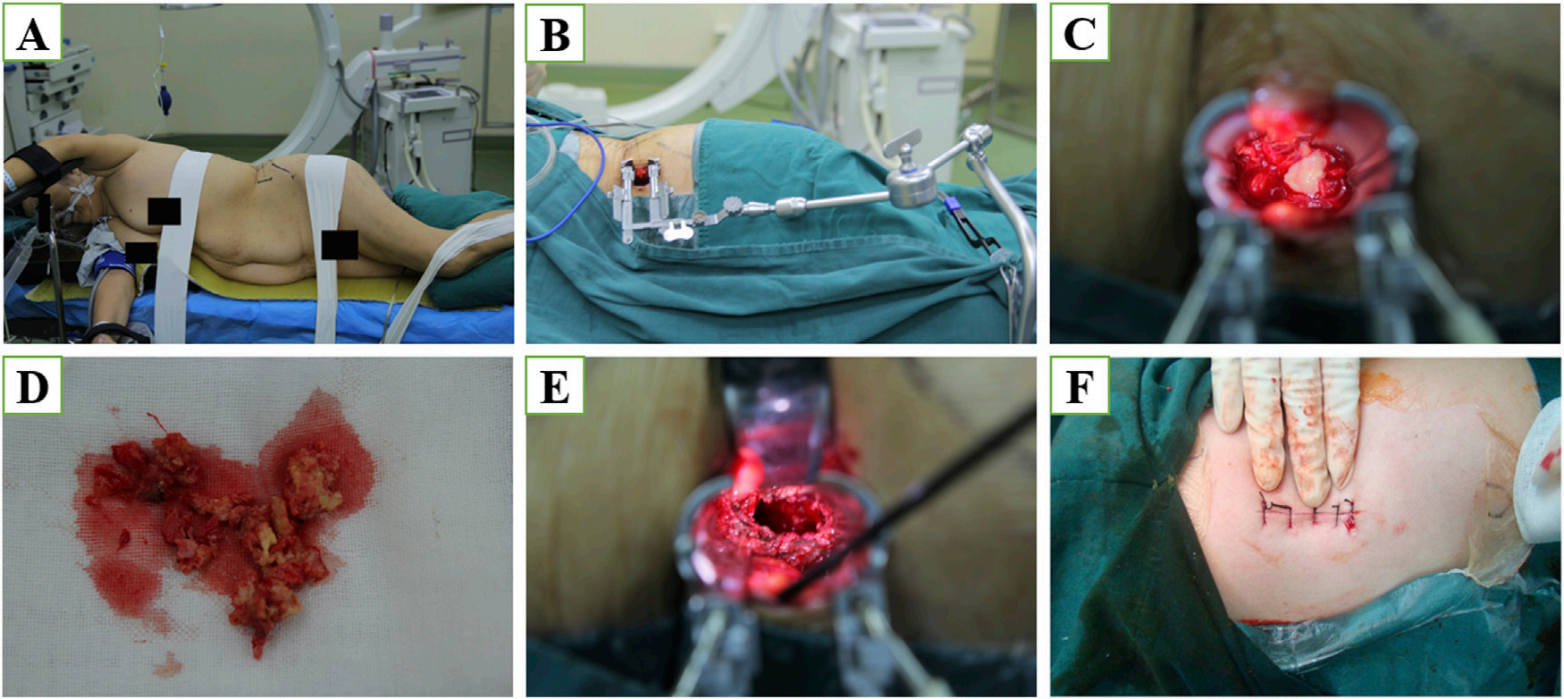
(A) Skin marking to check the disk level using a C-arm X-ray imager. A longitudinal skin incision was made 5–7 cm anterior to the front of the centrum (a minimum of 5 cm is recommended). The peritoneum was cleared from the psoas using blunt dissection. (B) Sequential dilation was used to place a working channel. (C) Abscess secondary to lumbar spinal tuberculosis. (D) Dead bone and a small amount of abscess tissue were removed. (E) The remaining unaffected vertebral body was used as a graft bed. (F) The width of the incision is only three fingers.
